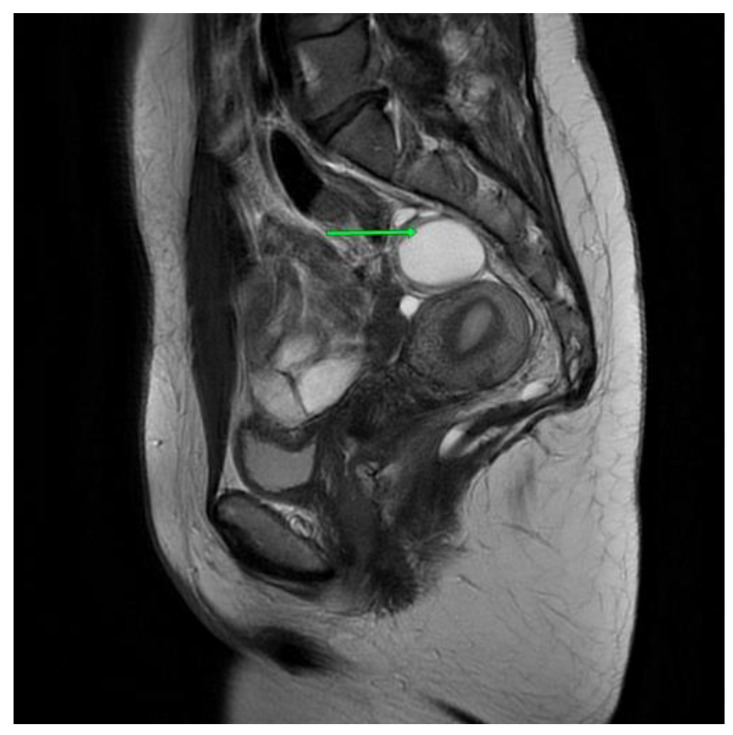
Second patient MRI scan of lesser pelvis. Green arrow shows the cyst.
